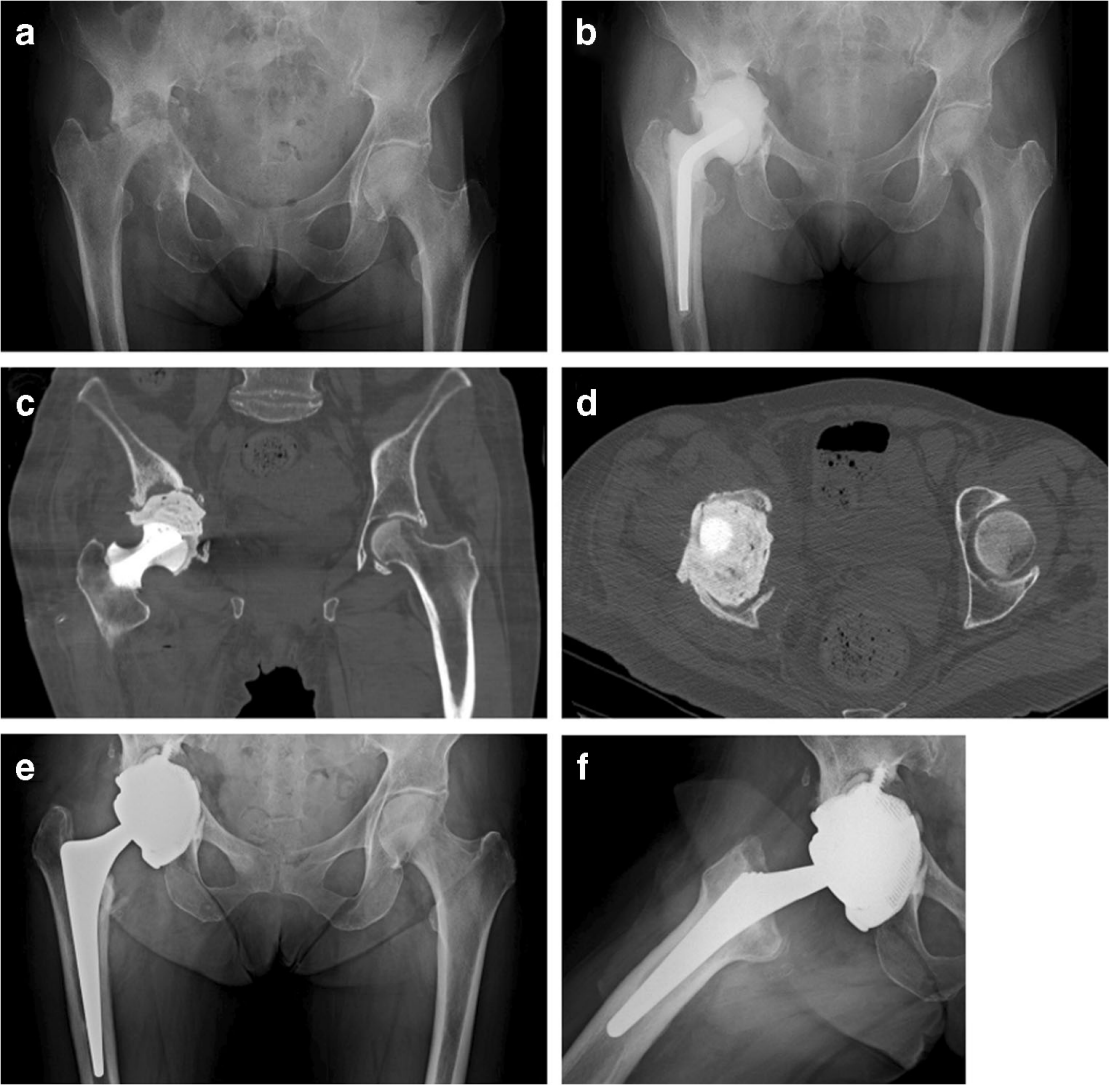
Images of a 76-year-old female patient suffering from polymicrobial SA of the right hip. a AP view of the pelvis showing Paprosky type IIIB acetabular bone defect and Paprosky type I bone defect on the femur. b AP radiograph of the pelvis 2 months after articulating cement spacer implantation. On the femur, a prefabricated cement spacer was used, whereas a hand-molded acetabular spacer was used on the acetabular side. c, d AP and axial CT details of acetabular bone defect. e, f AP and lateral views of the right hip at final follow-up. A single wedge cementless stem was used, and the extensive acetabular defect was managed through a custom-made component
